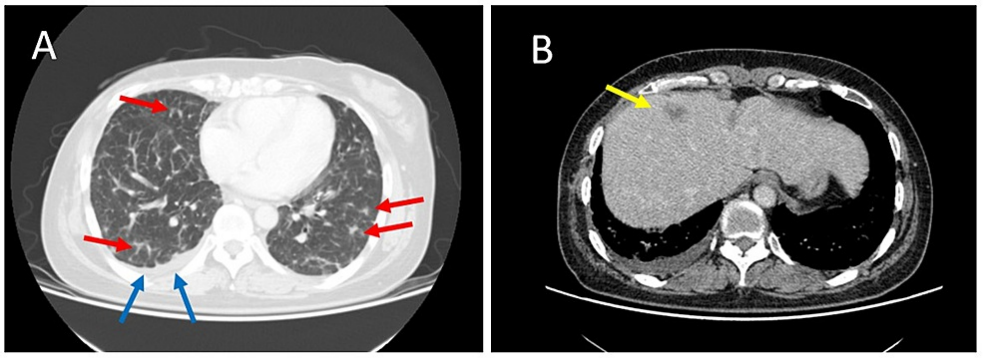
CT scan images. (A) The red arrows indicate multiple lung metastases, and the blue arrows indicate pleural effusion. (B) The yellow arrow indicates liver metastasis. CT: computed tomography
